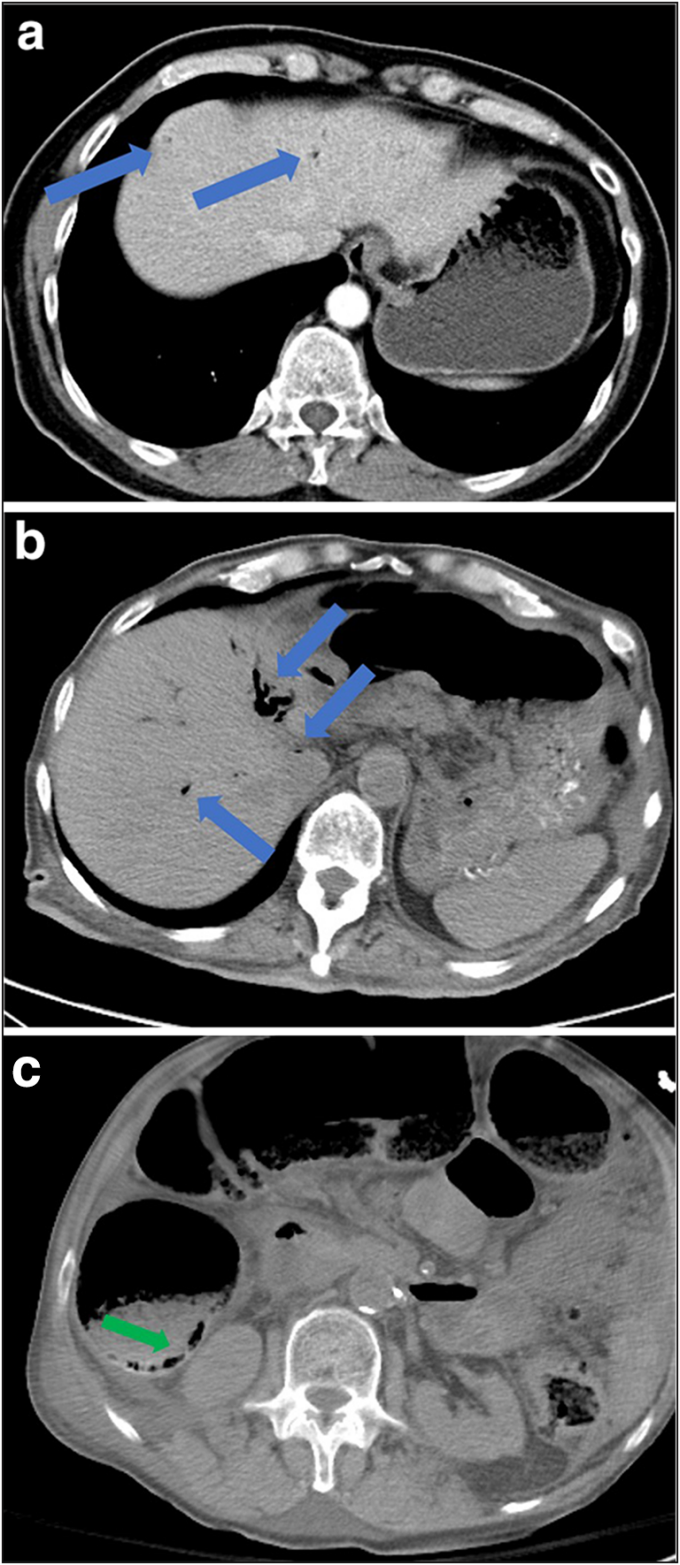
a, b Axial CT scan images demonstrate intraluminal air foci in the main portal venous systemic vessels and peripherally in the intrahepatic vessels (blue arrows). c Axial CT scan demonstrating a pneumatosis intestinalis (green arrow).
